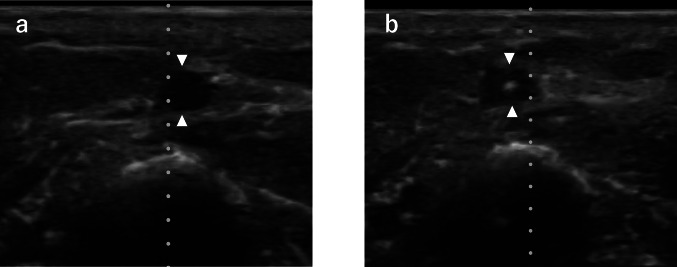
Ultrasound images acquired through the film in a clinical setting. a Transverse image of the target vein for puncture: arrowheads indicate the vessel. b Transverse image of the vein during puncture: arrowheads indicate the vessel, and the bright spot within the vessel is the needle tip
